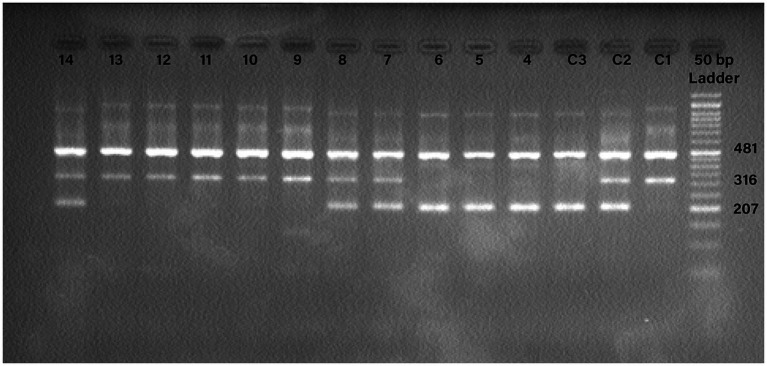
Detection of the PCR products for TMPRSS2 rs2070788 polymorphism. Internal control band 481 base pair (bp) is found in all wells, with band 207 bp representing allele C and band 316 bp representing allele T. C1: control TT; C2: control CT; C3: control CC; 4, 5, 6: CC; 7, 8, 14: CT; 9, 10, 11, 12, 13: TT.
